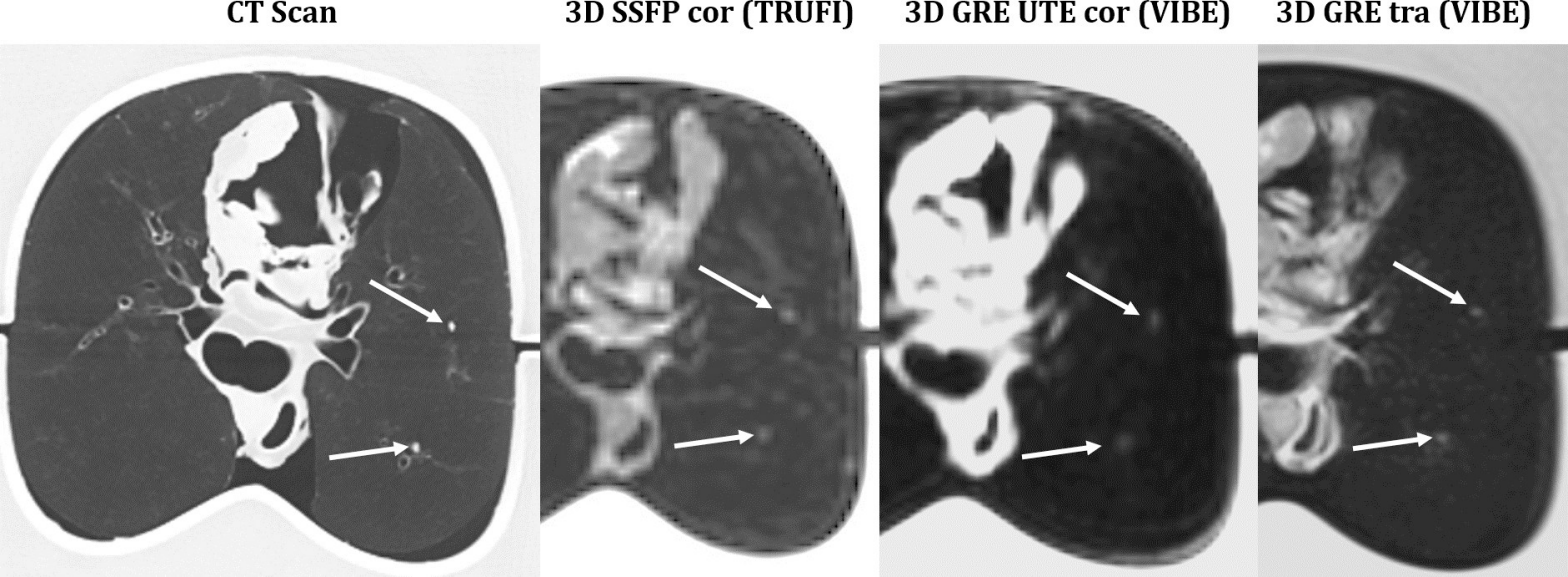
CT-MRI comparison of 2 nodules with a diameter of 2mm.
